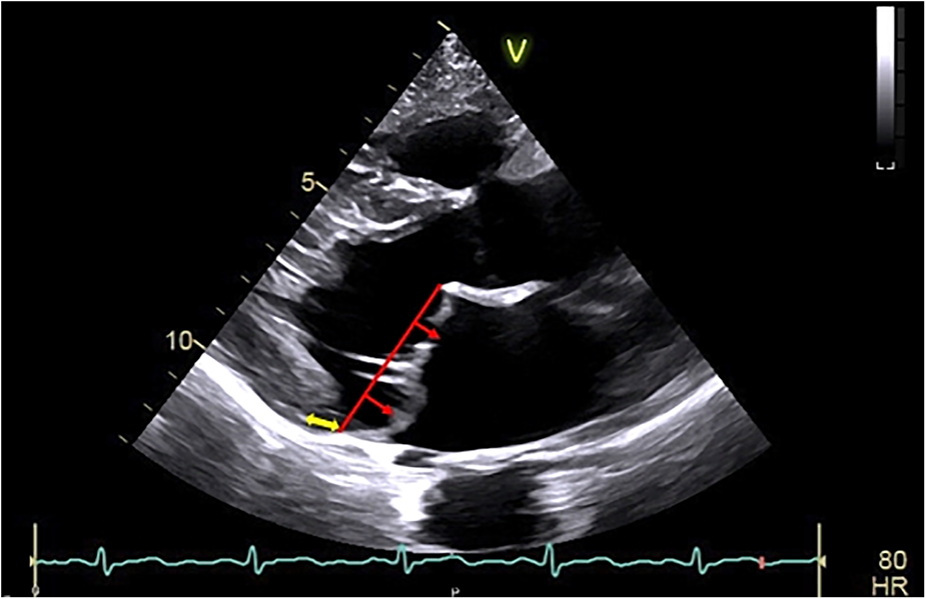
Arrhythmic mitral valve prolapse by transthoracic-echocardiography. Transthoracic-echocardiographic long-axis view in end-systole with a displacement of both leaflet >2 mm (red arrow) above the plane of the annulus (red line) defining a bileaflet mitral valve prolapse with thick leaflets linked to myxomatous degeneration. Note the presence of the detachment of the posterior leaflet from the left-ventricular myocardium (mitral annular disjunction, yellow arrow) to be assessed in dynamic analysis (that is a frame by frame analysis during the entire cardiac cycle) to better ascertain the position of the posterior mitral annulus.
